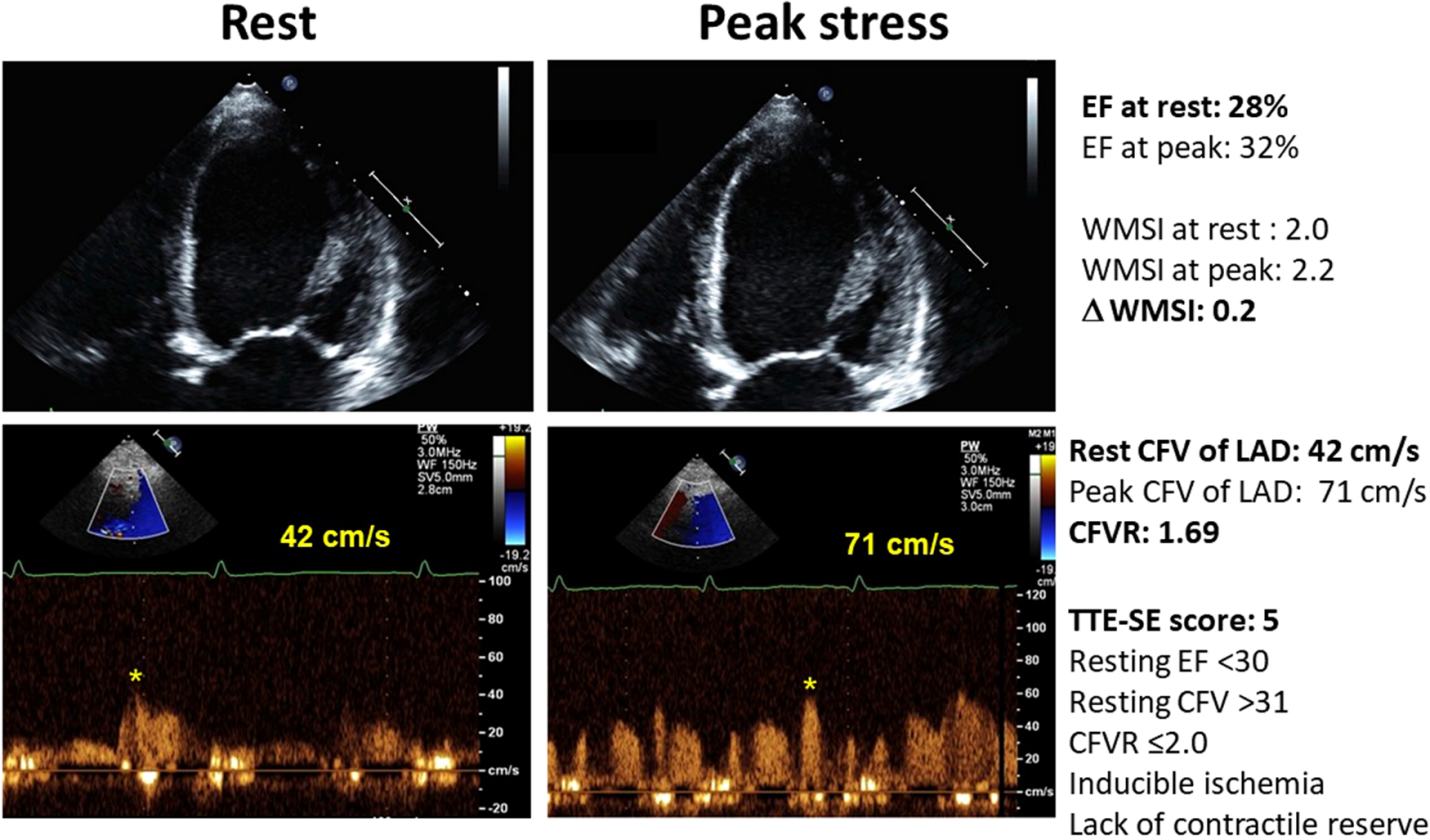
The abnormal pattern of high resting flow velocity with reduced CFVR with dipyridamole stress, with unchanged LV end-systolic volumes and no regional wall motion improvement during stress. Upper panel, color-Doppler signal; lower panel, pulsed-wave Doppler signal.
